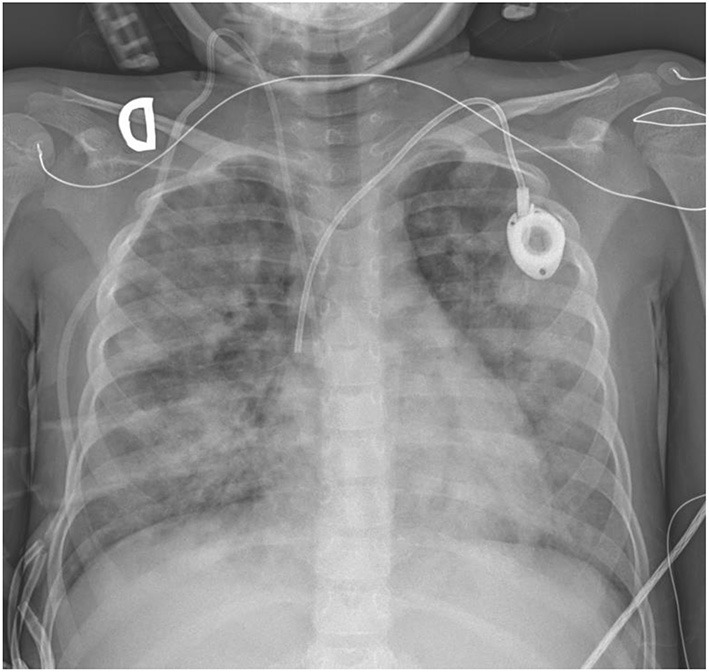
Chest radiography on admission.
